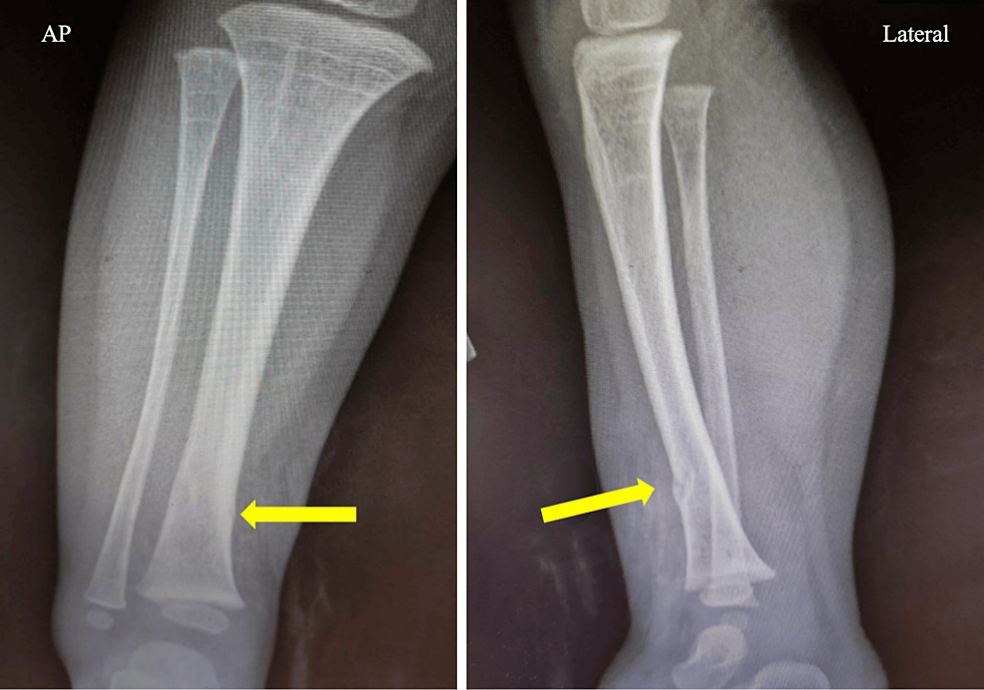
AP view X-ray imaging of the right leg The arrows point to the distal tibial shaft and distal fibula fractures
